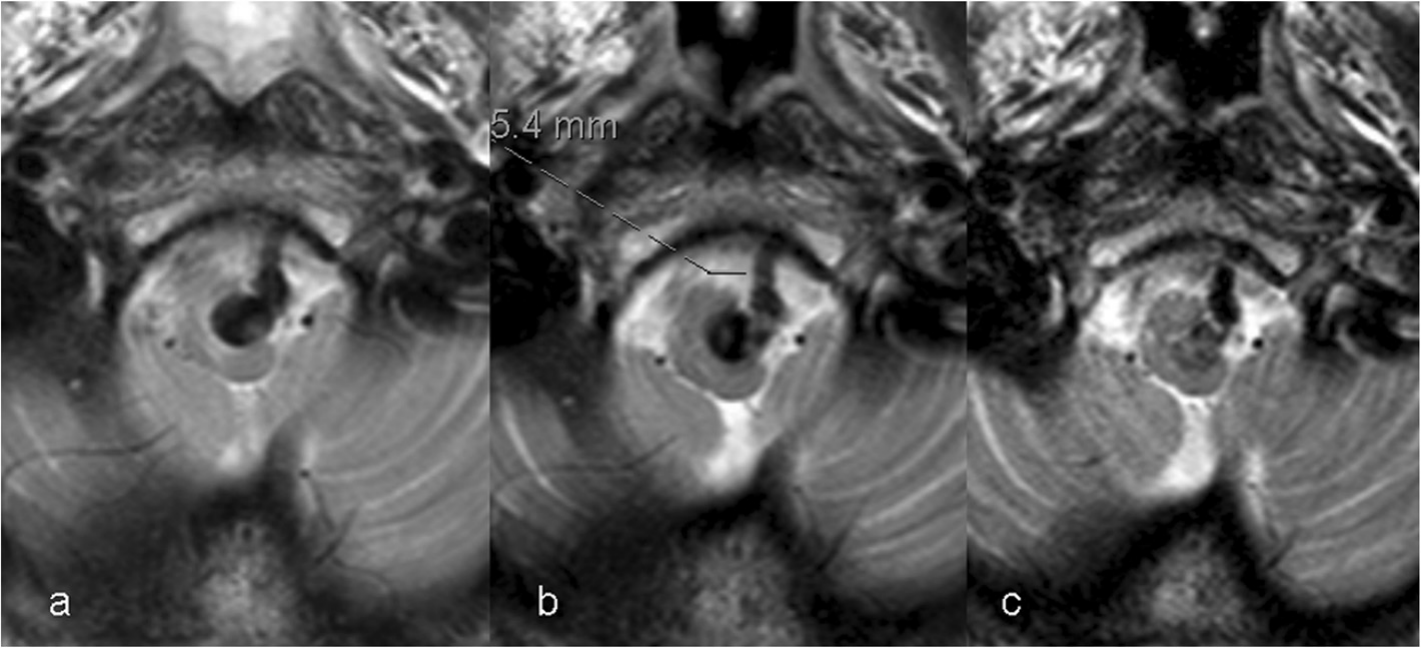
Gradual shrinkage of the aneurysm during the follow-up period: T2-weighted MR performed (a) prior to treatment, (b) at 4-month follow-up, and (c) 1 year after the treatment show that the aneurysm is diminished in size and the volume of the subarachnoid space around the medulla has been almost completely normalized.
